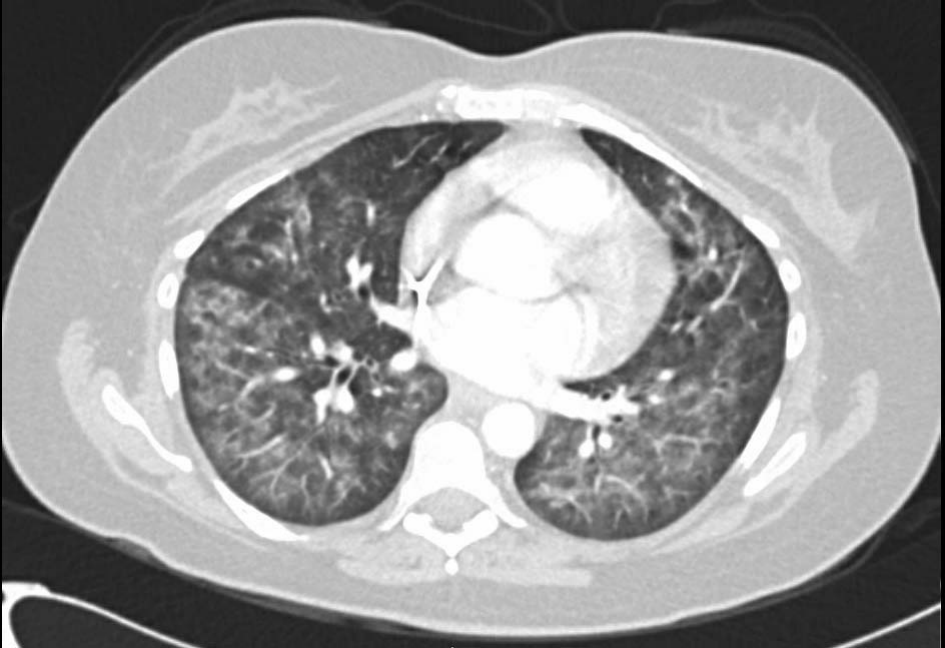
Case 2: Computed tomography angiogram of the chest shows extensive interstitial and airspace opacities.
